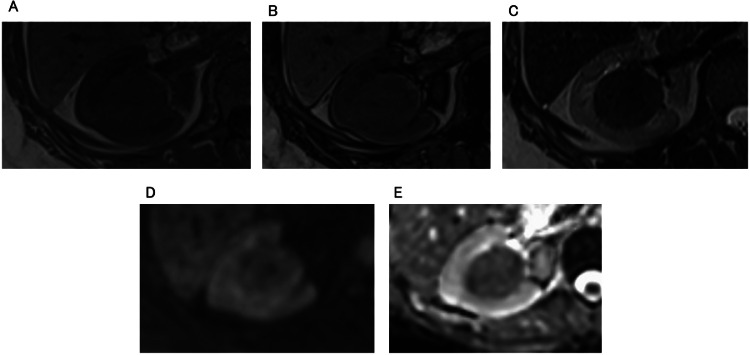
MRI. (A) T1 in-phase images, (B) T1 out-of-phase images, (C) T2WI, (D) diffusion-weighted image (DWI), and (E) apparent diffusion coefficient (ADC) map. Tumor demonstrates a homogeneous low signal on T1W (A). There was no low signal indicative of a small amount of fat on T1 out-of-phase images (B). On T2WI, a homogeneous low signal is observed (C). ADC value is 1.27 × 10^-3^ mm^2^/sec, demonstrating no significant diffusion restriction (D, E).
